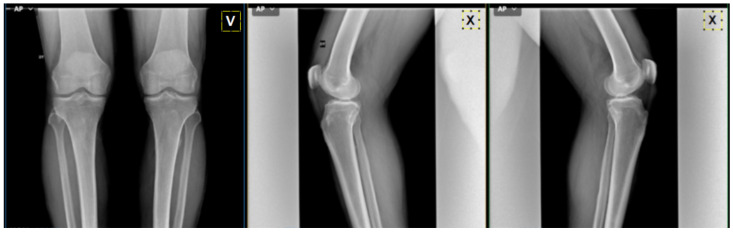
Example of knee X-ray images collected and filtered from patients with knee symptoms.
